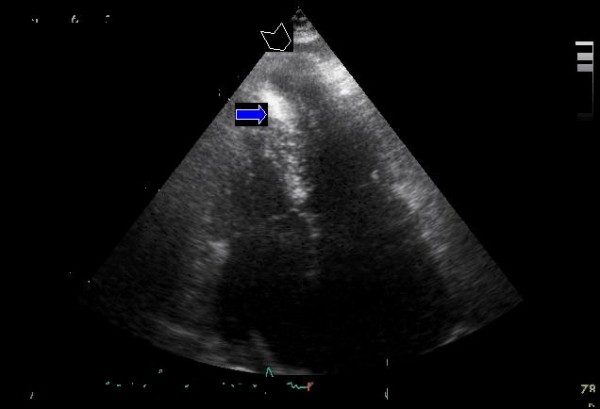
Two dimensional transthoracic echocardiogram. Apical four chamber view showing predominant thickening of midseptal and midlateral regions during systole (blue arrow) and the formation of left ventriculat apical aneurysm (arrow head) in case index-1 patient.
